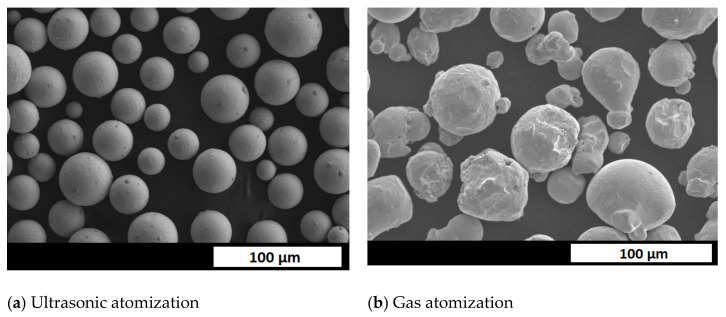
SEM images of <50 µm (a) ultrasonically atomized powder (b) gas-atomized powder.
